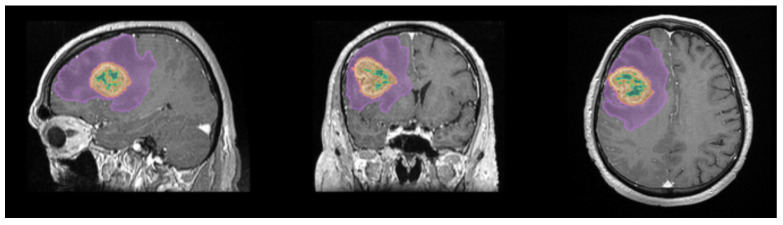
Mdbrain software processing of MRI brain acquisition (DICOM): the segmented regions include the necrotic core (green), the contrast-enhancing tumor component (orange), and the perilesional edema (purple) (approval was obtained from the Ethics Committee of the University of Medicine and Pharmacy “Grigore T. Popa” Iasi).
